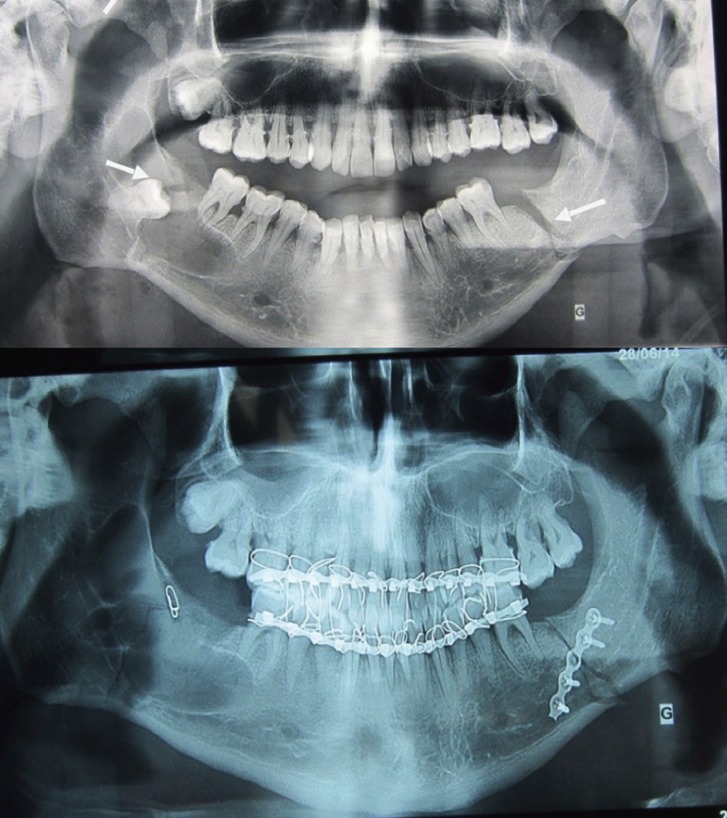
Orthopantogram and CT scan showing bifocal mandibular fracture and mandibular cyst. Orthopantogram shows an evident fracture of the left mandibular rim and cystic cavity in the right side. Orthopantogram after the surgery.
